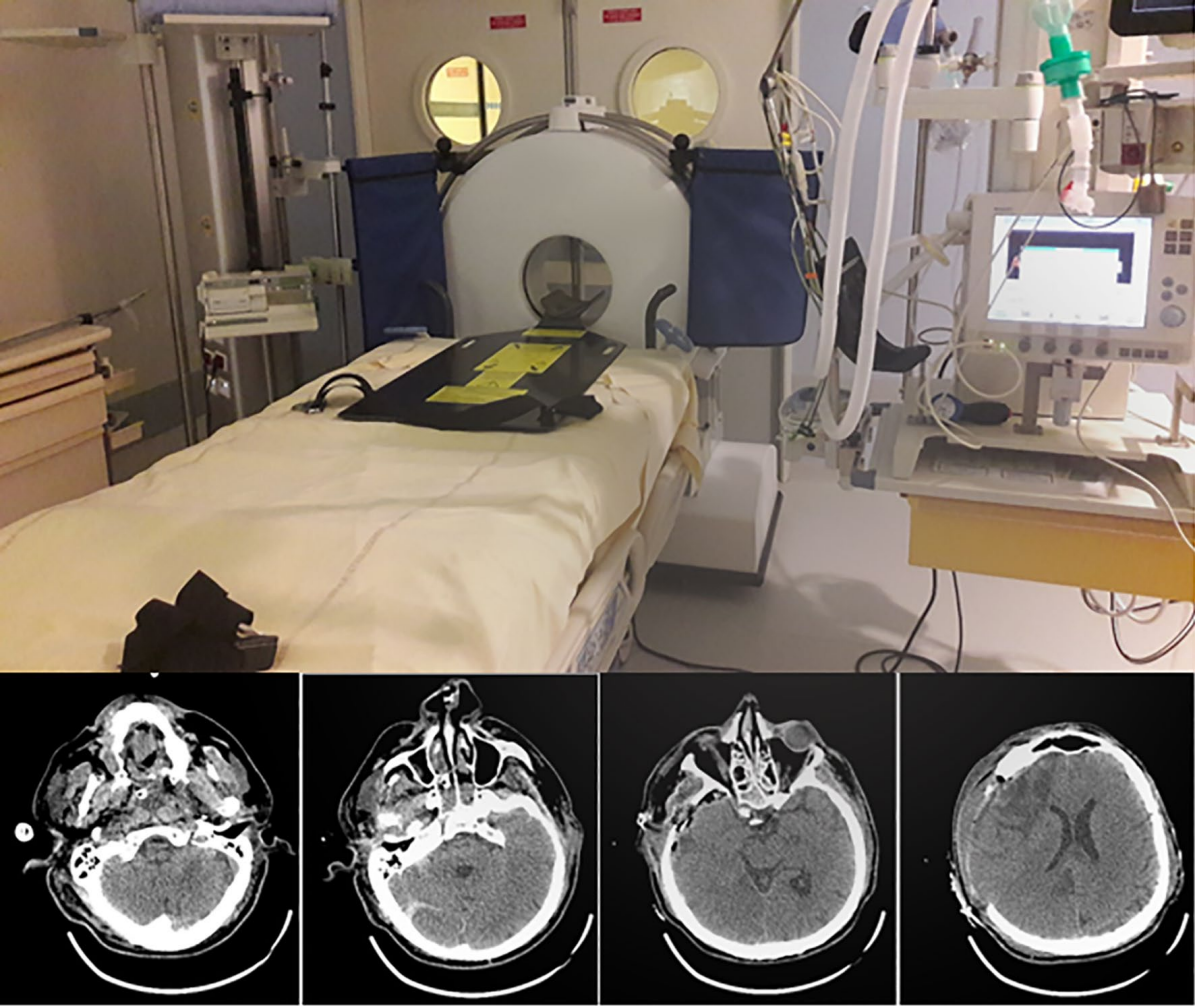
Position of the Ceretom portable CT scanner in an intensive care unit room and 4 slices from a non-contrast pCTH examination.
